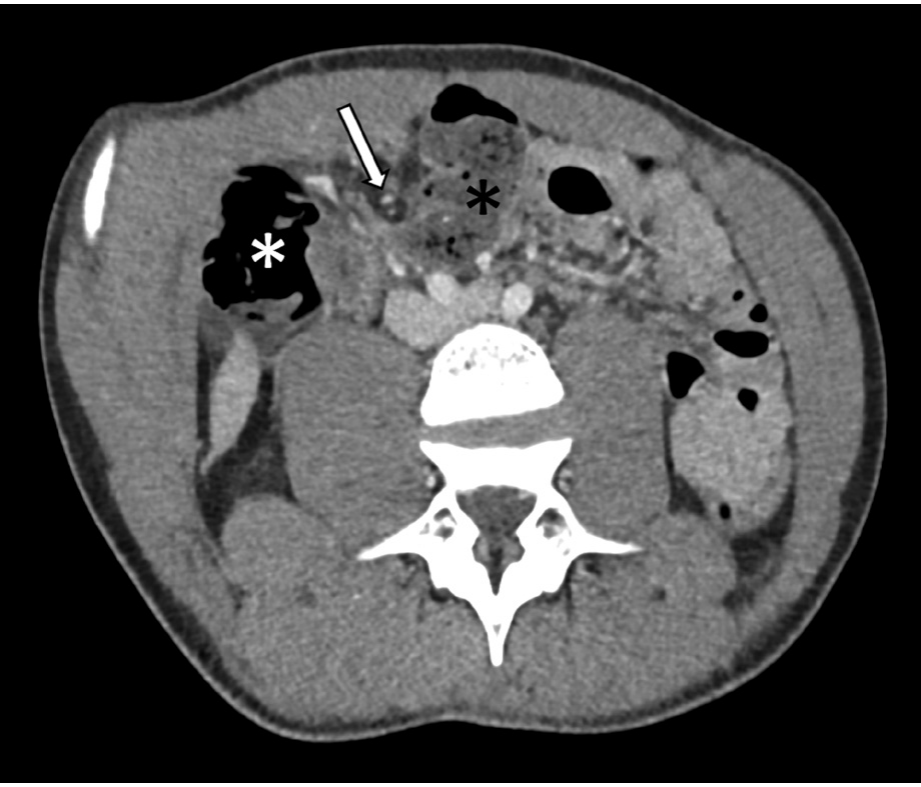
Axial/oblique image from a contrast enhanced CT exam showing feces-like material within the ileum (black star) with a transition point in the right iliac fossa around a fibrous band (arrow). The colon is on the other side of the transition point (white star).
